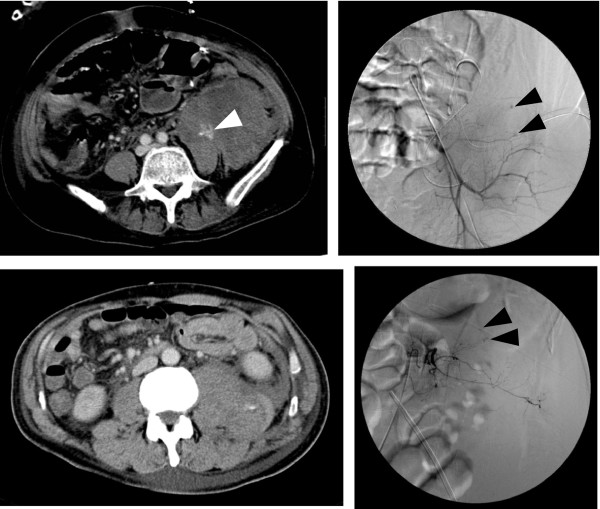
Computed tomography and angiographic images in cases 1 and 2. Upper left panel shows abdominal CT image from case 1. A giant intramuscular hematoma in the iliopsoas muscle and the active bleeding point (white arrowhead) are shown. Upper right panel shows an angiographic image from case 1. Extravasation was found in the superior gluteal artery area (black arrowheads). Lower left panel shows an abdominal CT image from case 2. A left iliopsoas muscle hematoma is shown. Lower right panel shows an angiographic image from case 2. Multiple extravasations were found in the 3rd lumbar artery (black arrowheads).
